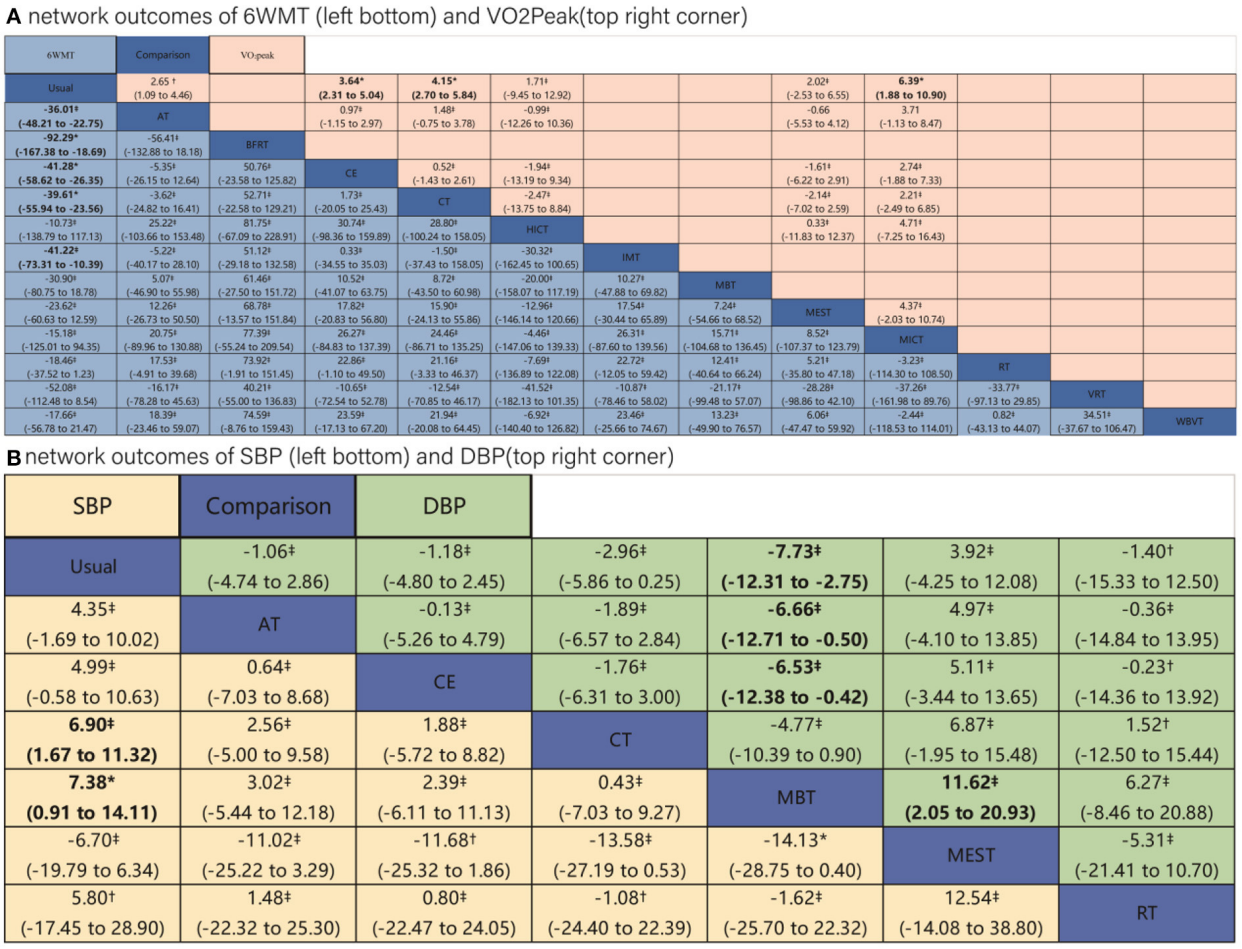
Results of network meta-analysis of four outcomes. Comparisons should be read from left to right. (A) Outcomes of 6 WMT and VO_2_Peak. (B) Outcomes of SBP and DBP. Outcomes are located at the intersection between the column-defining treatment and the row-defining treatment. Data are in MD (95% CIs). For 6MWT and VO_2_Peak, MDs above 0 favor the column-defining treatment. For SBP and DBP, MDs below 0 favor the column-defining treatment. The certainty of the evidence [according to confidence in network meta-analysis (CINeMA)] was incorporated in this figure as footnotes. ^*^Moderate quality of evidence. ^†^Low quality of evidence. ^‡^Very low quality of evidence. The significant results in league tables have been bolded. AT, aerobic training; Usual, usual care; CT, combined training; RT, resistance training; CE, cycle dynamometers; MEST, electrical muscle stimulation; IMT, inspiratory muscle training; BFRT, blood flow restriction exercise; HICT, high-intensity circuit training; MICT, medium intensity continuous training; VRT, virtual reality training; MBT, body and mind training.
